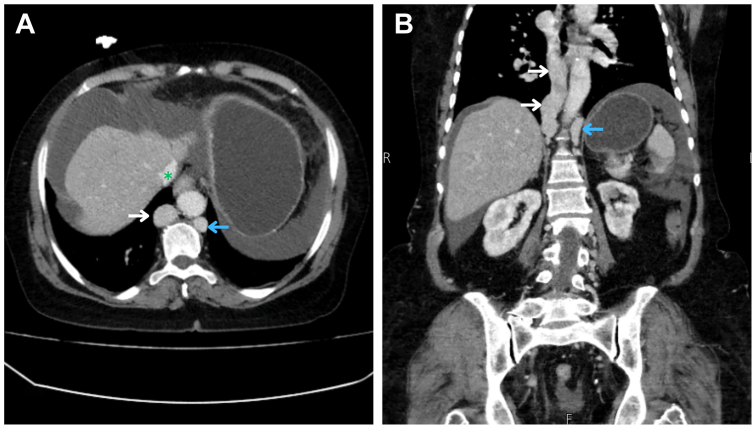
Contrast-enhanced axial (A) and coronal (B) computed tomography angiography (CTA) images show dilated azygous (*white arrow*) and hemiazygos (*blue arrow*) veins, with a normal-caliber supra-hepatic inferior vena cava (IVC; *green asterisk*, axial image [A]).
